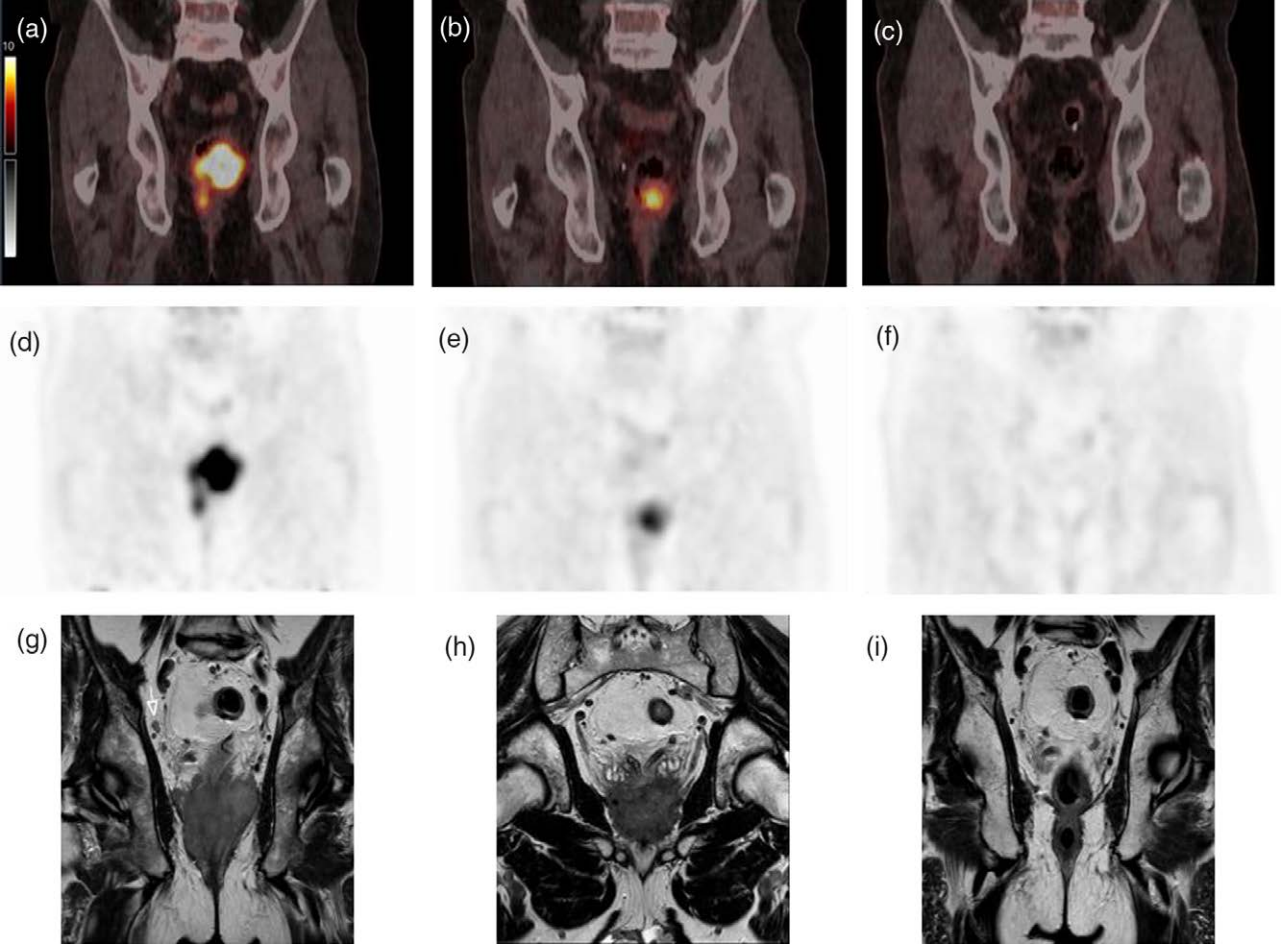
[^18^F]FDG PET/CT and T2 weighted MRI images before, during, and after neoadjuvant therapy of a patient with clinical complete response. A sixty-two-year-old man with cT4bN2M0 rectal cancer had a good response to a yiT1-2N0M0 which further regressed to a yiT0N0M0 6 months after chemoradiotherapy, and is currently still followed in the watch-and-wait after 12 months of recurrence-free follow-up. SUV_max_ was 18.1 at baseline, 10.4 at interim assessment, and too low to measure at reevaluation. Figure shows [^18^F]FDG PET/CT fusion (a–c) and PET-only (d–f) images as well as T2 weighted MRI (g–i) images before (a, d, g), during (b, e, h) and after (c, f, i) neoadjuvant chemoradiotherapy. CT, computed tomography; SUV_max_, maximum standardized uptake value.
